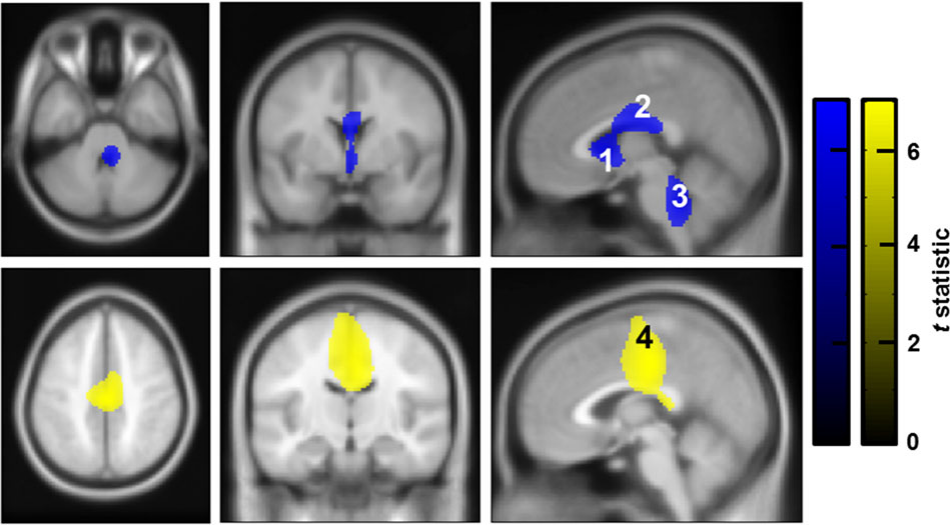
Brain regions with significant coherence with the PPNR. Frequency-specific whole brain images of coherence with the PPNR (DICS images) were entered into an ANOVA with surrogate shuffled data. Note that all images from left-sided PPNR-LFP data have been reflected across the median sagittal plane to allow inference regarding ipsilateral versus contralateral areas of coherence. The resulting thresholded SPMs are displayed above, overlayed onto corresponding orthogonal sections through an averaged T1 weighted MRI in MNI space. Each significant cluster has been labeled with a corresponding number, which corresponds to the ROI label. Top row (blue): alpha coherence with the PPNR was greater than surrogate coherence in the subgenual cingulate (ROI 1), the posterior midcingulate (ROI 2) and the posterior brainstem (ROI 3). Bottom row (yellow): Beta coherence with the PPNR was significantly greater than surrogate coherence in the medial frontal wall in an area that overlapped with the posterior midcingulate, the SMA and the leg area of the primary motor cortex (ROI 4). Corresponding peak location statistical data are presented in Table 2. The color bar represents the *t* statistic for alpha (blue) and beta (yellow) coherence. Images are thresholded to *P* < 0.05 (FWE corrected).
